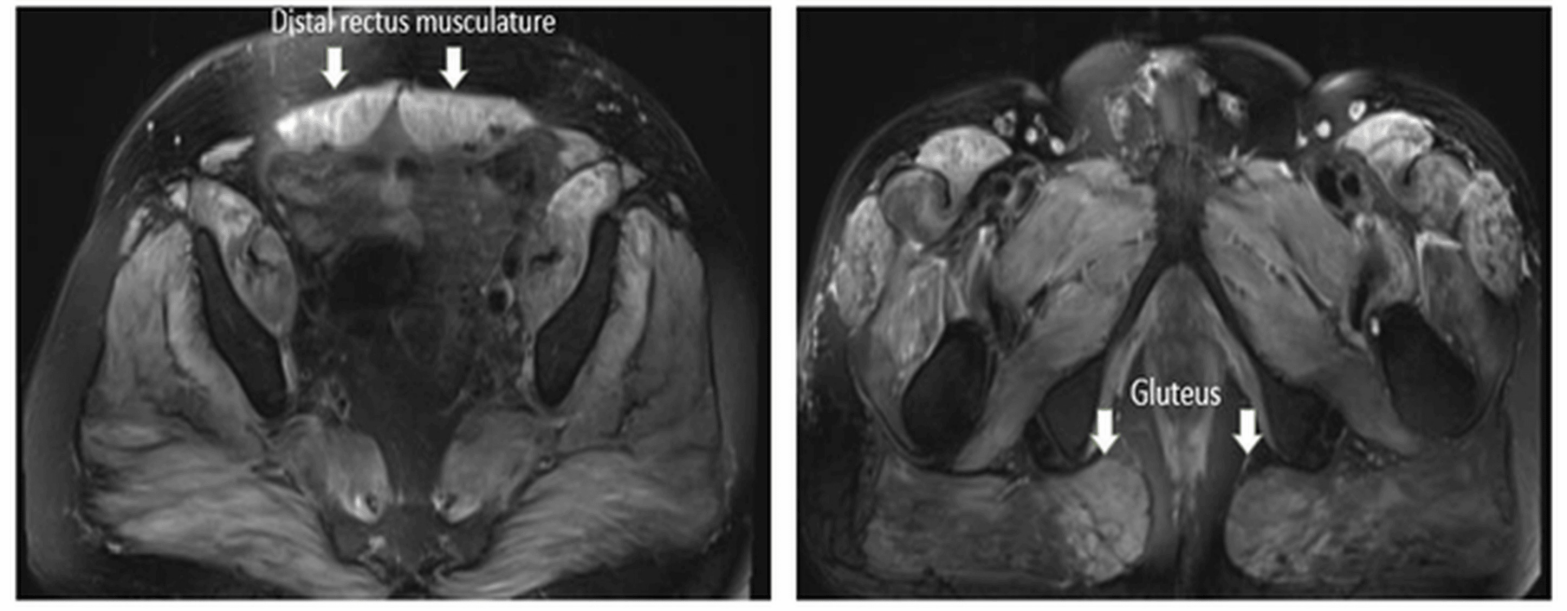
MRI of the lower extremity without contrast - T2 hyperintensity of parapelvic musculature
